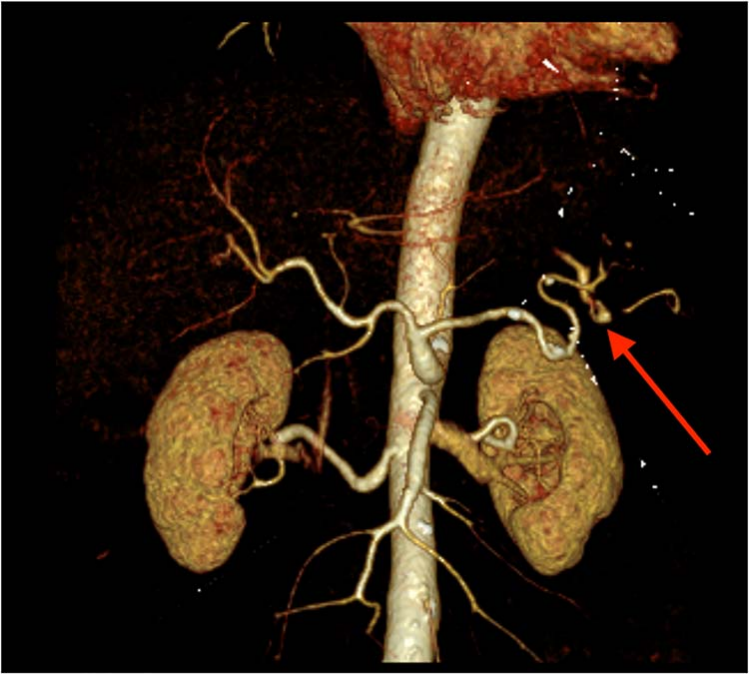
CT angiography of the abdomen with 3D reconstruction. Ruptured SAP.
